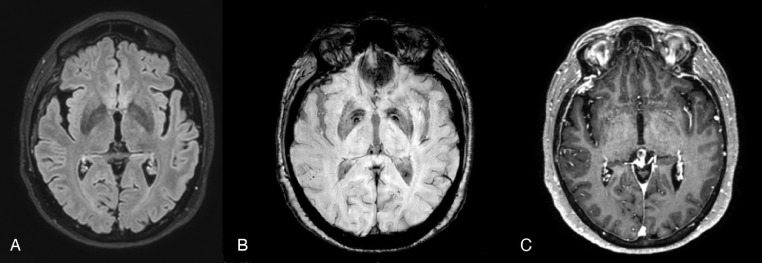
CASE 2 (A) FLAIR ax; (B) SWI ax; post contrast T1 MPRAGE ax. The first MRI study showed a little cluster of cortical-subcortical hypointense foci on Susceptibility weighted imaging (SWI) (B) sited in the right superior temporal gyrus and near the angular gyrus, without abnormal signal of the surrounding white matter on FLAIR images (A). No enhancement was seen (C).
